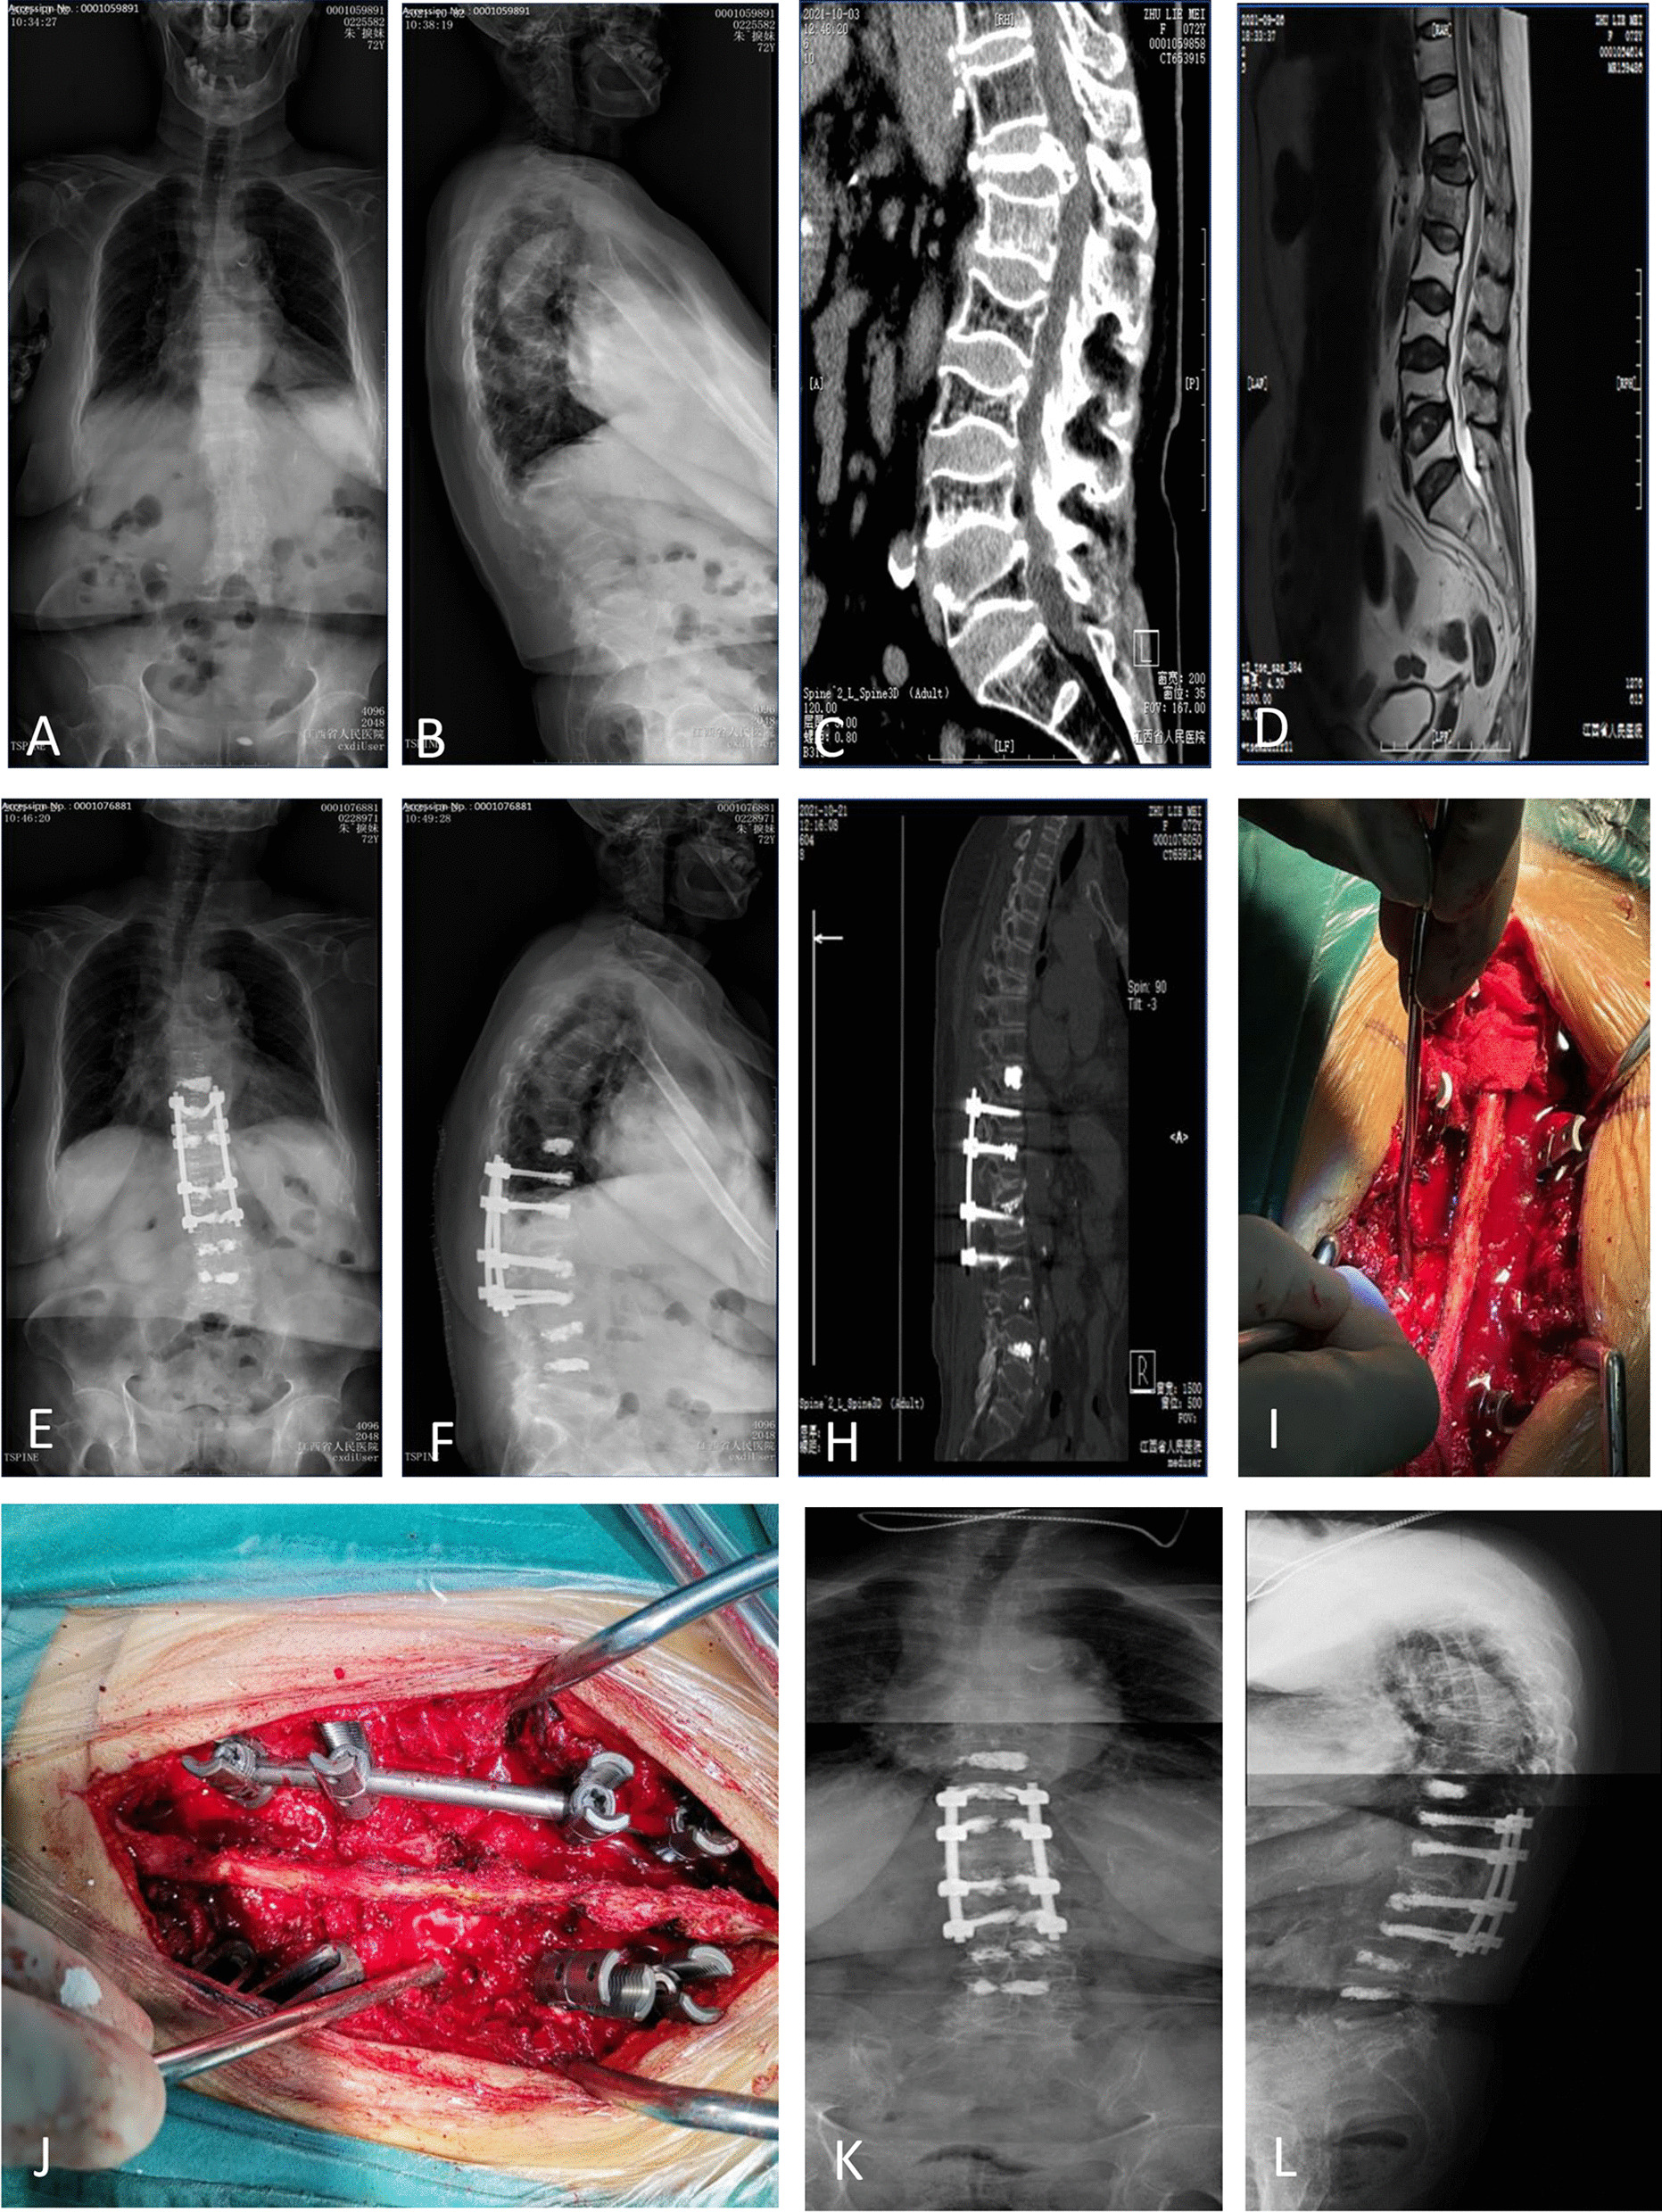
A 72-year-old female patient was admitted for low back pain and weakness in both lower limbs. Physical examination revealed local kyphosis, Franke grade D, and no pathological signs. BMD: − 5.7, VAS: 6 points, ODI: 75. T12 fracture with vertebral collapse, kyphosis deformity, RKA: 35.4°, SCCr: 54.8, AVHr: 25.9 (A, B). 3D CT of the spine showing a wedge-shaped collapse of the T12 with protruding bone blocks entering the spinal canal from the posterior wall (C). MRI of the thoracolumbar region shows significant compression of the spinal cord at T12 (D). Lumbar spine radiographs in both anteroposterior and lateral views. The patient underwent local laminectomy at T12 and spinal internal fixation at T10-L2, with some vertebral bodies reinforced with bone cement. Postoperative 1-week RKA: 21.7°, SCCr: 77.1, AVHr: 58.0 (E, F). Final follow-up, RKA: 25°, SCCr: 66.8, AVHr: 49.3 (K, L)
